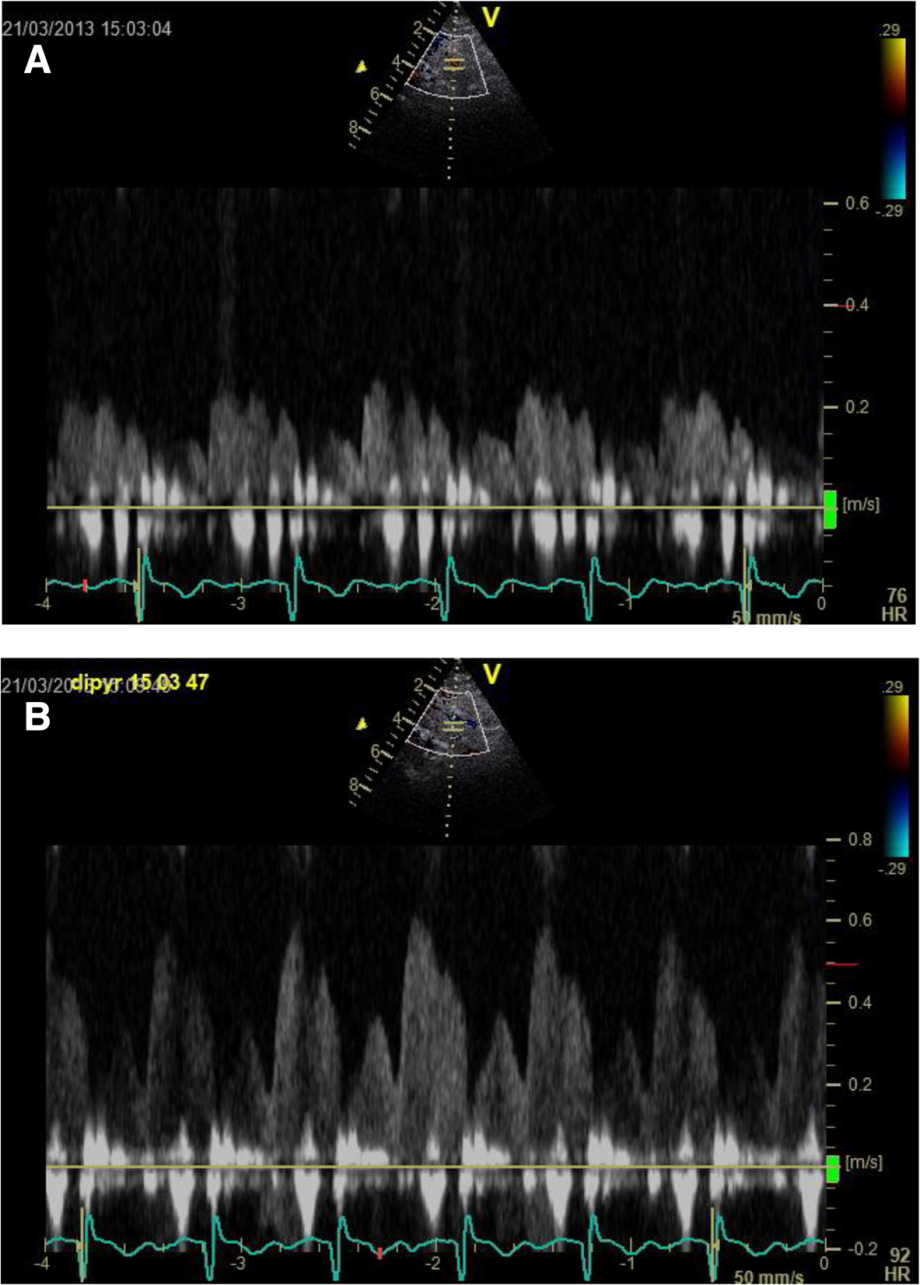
Measuring CFR by Doppler flow echocardiography. Left anterior descending artery (LAD) flow during rest (A) and LAD flow during dipyridamole induced stress (B).
